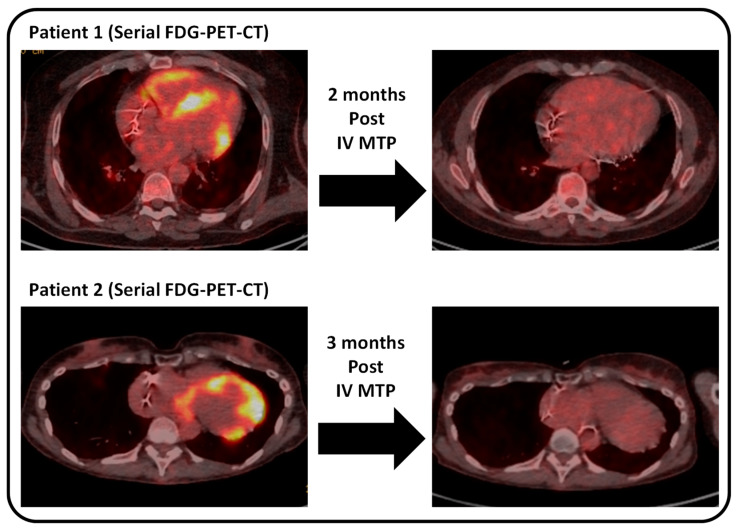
Immunosuppressive treatment response in two patients with cardiac sarcoidosis and active myocardial inflammation on [^18^F]fluorodeoxyglucose positron emission tomography integrated with computed tomography (FDG-PET-CT). Patient 1 had intense FDG uptake in the left and right ventricles, which resolved on repeat FDG-PET-CT 2 months after intravenous (IV) methylprednisolone (MTP) therapy. Patient 2 had intense FDG uptake in the left ventricle, which also resolved on repeat FDG-PET-CT 3 months after IV MTP therapy.
